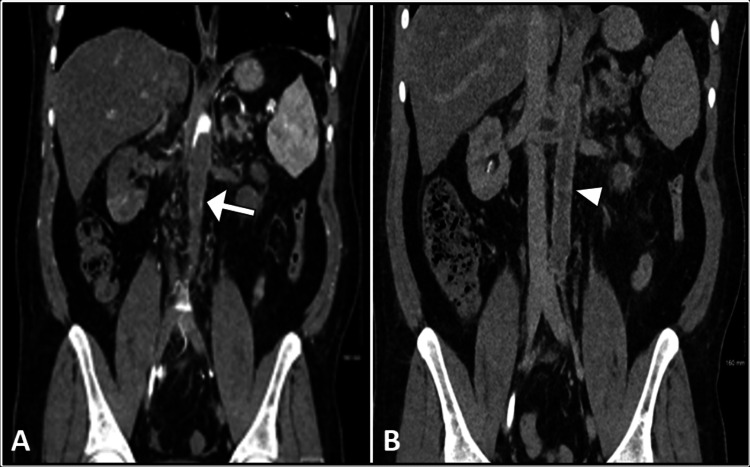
Coronal CT images show filling defects in the infrarenal abdominal aorta (arrow) in the portal venous phase (A). Enhancement of the abdominal aorta (arrowhead) is noted in the delayed phase (B). CT: computed tomography
